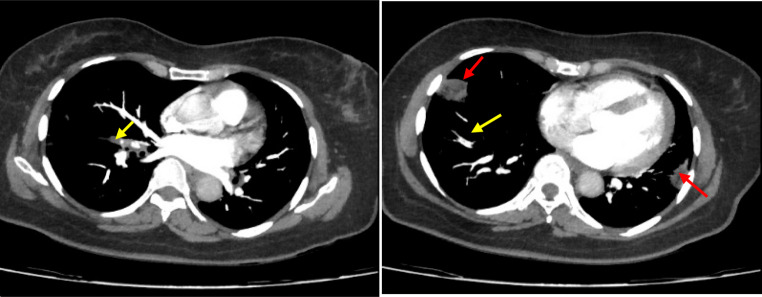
CT pulmonary angiography revealing a segmental filling defect in the right pulmonary artery (yellow arrow) and a subsegmental defect in the left (blue arrow), with associated bilateral infarctions (red arrows)
